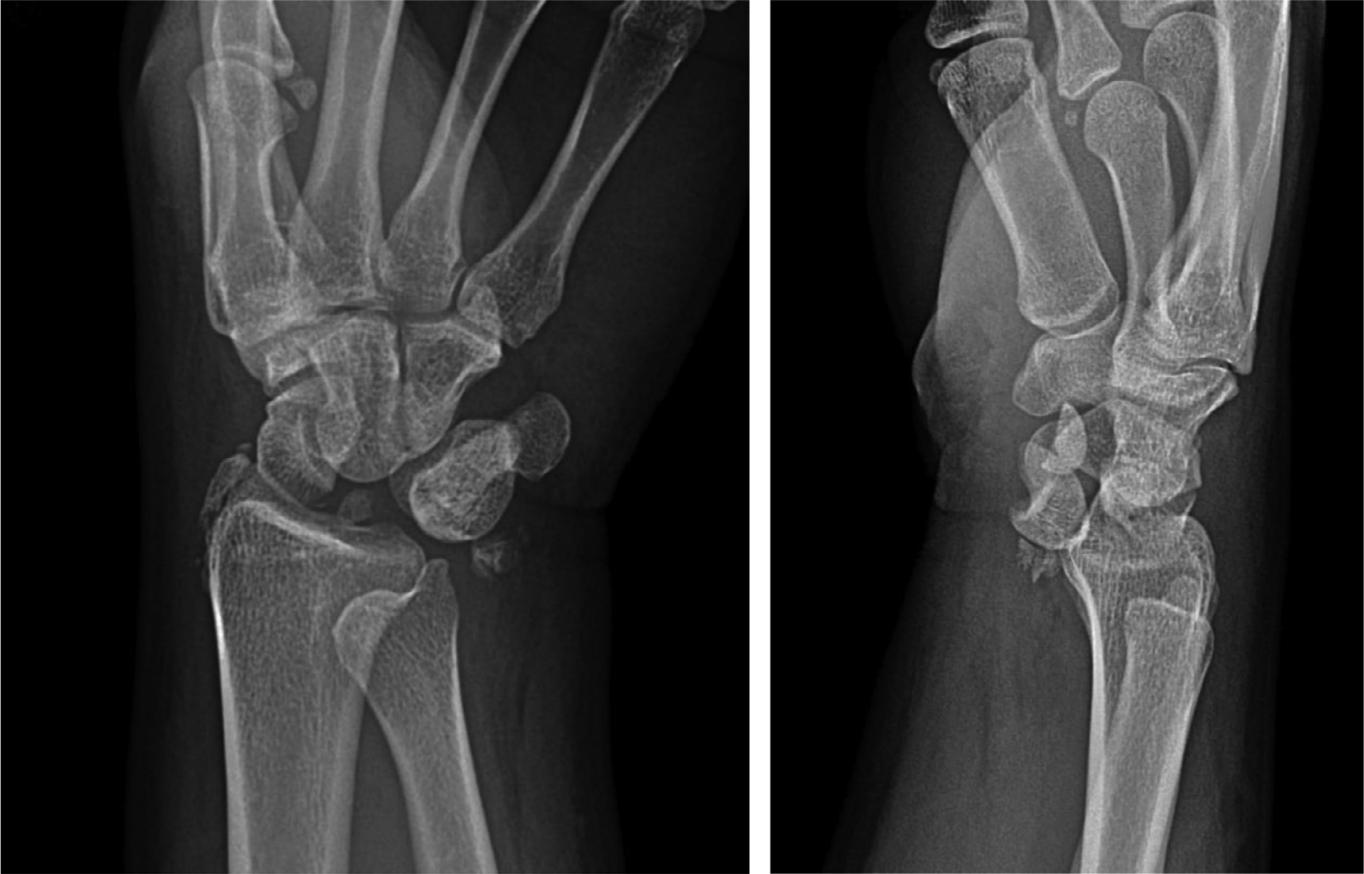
Patient 4: Initial radiographs of the right wrist reveal a trans-radial styloid trans-scaphoid lunate dislocation. Irregular fracture fragments at the ulnar volar aspect of the wrist may be from the scaphoid or pisiform. The radial styloid fracture is nondisplaced. There is negative ulnar variance.
